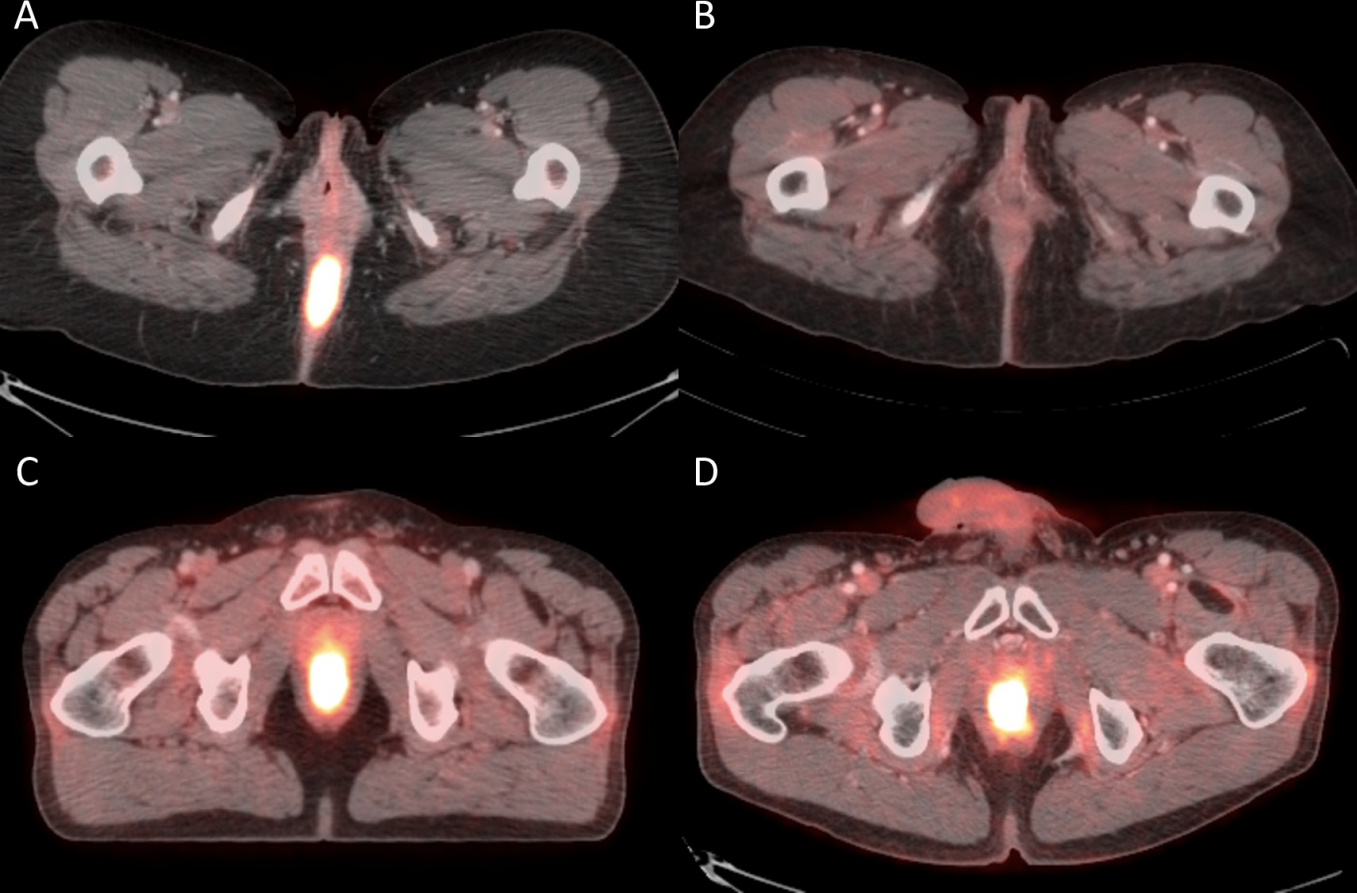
Pre and post-treatment PET/CTs for a patient without (patient 1) and with (patient 2) local recurrence A) Pre-treatment PET/CT of patient 1 with MTV_2.5_ of 49.65cc (B) Post-treatment PET/CT of patient 1 with MTV_2.5_ of 6.21cc C) Pre-treatment PET/CT of patient 2 with MTV_2.5_ of 41.44cc (D) Post-treatment PET/CT of patient 2 with MTV_2.5_ of 21.52cc.
